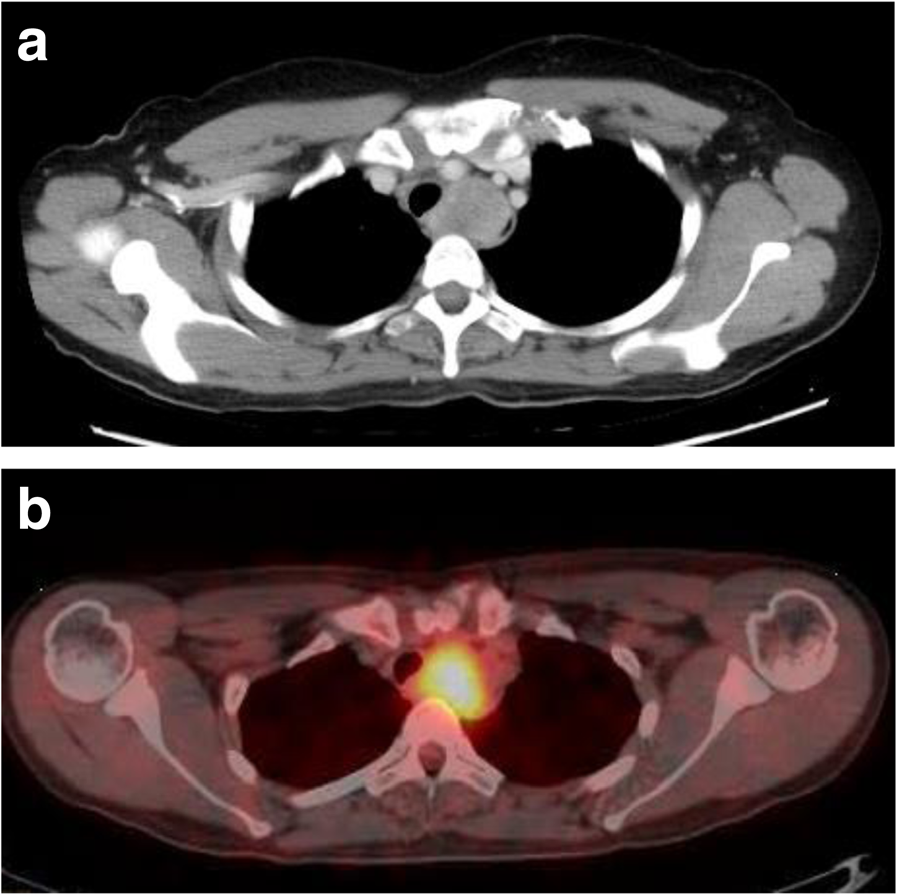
CT and FDG-PET CT findings. a Computed tomography revealed a large isodense mass of the esophageal wall in the upper mediastinal space. b Accumulation of fluorodeoxyglucose is demonstrated in the upper thoracic esophagus
